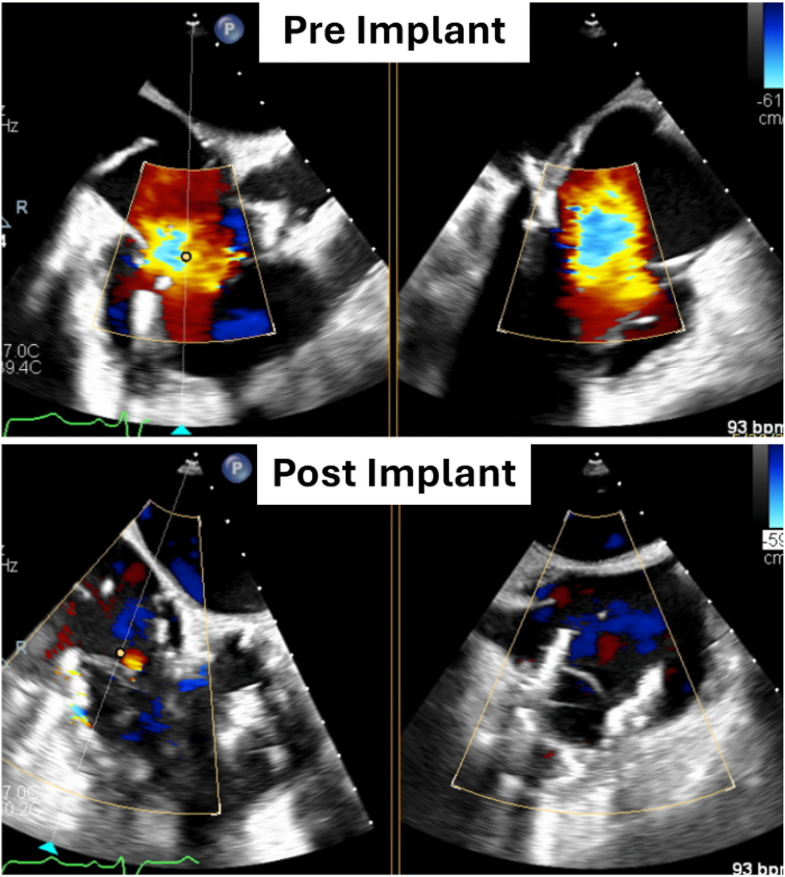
Transesophageal Echocardiography Before (Torrential Tricuspid Regurgitation) and After (None/Trace Tricuspid Regurgitation) Transcatheter Tricuspid Valve Replacement
